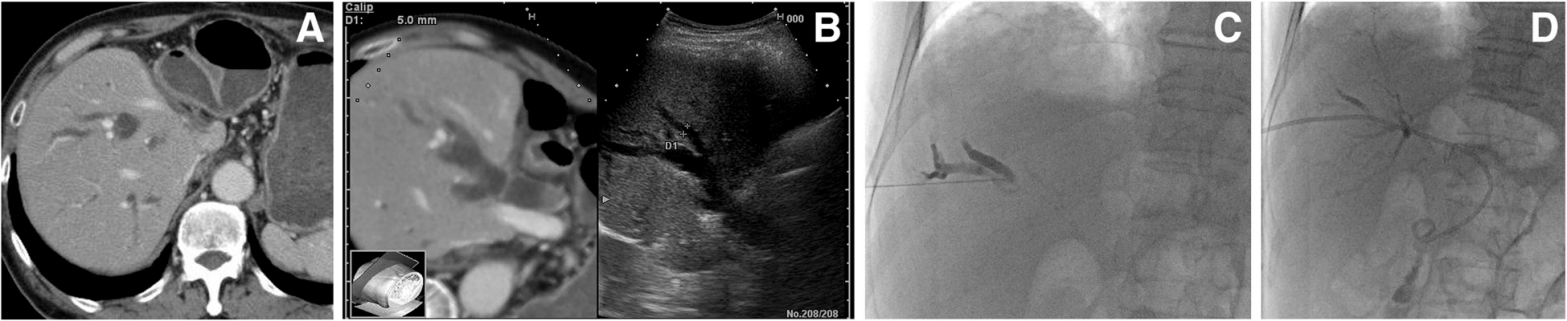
An 82-year-old man with jaundice due to lymphadenopathy of the porta hepatis from a sigmoid cancer. A: The CT image shows a dilated bile duct in the right liver lobe. The stomach cavity is in contact with the liver because the lateral hepatic segment was resected due to a liver metastasis. B: The virtual CT image and the B-mode sonogram show the dilated bile duct (B5: arrow). The virtual image shows that the stomach cavity (arrow head) is close to the liver; a B-mode sonogram cannot show this. C: The radiograph shows that the needle is safety inserted into the bile duct, avoiding the stomach as guided by the RVS system. D: The radiograph shows successful internal-external bile duct drainage.
